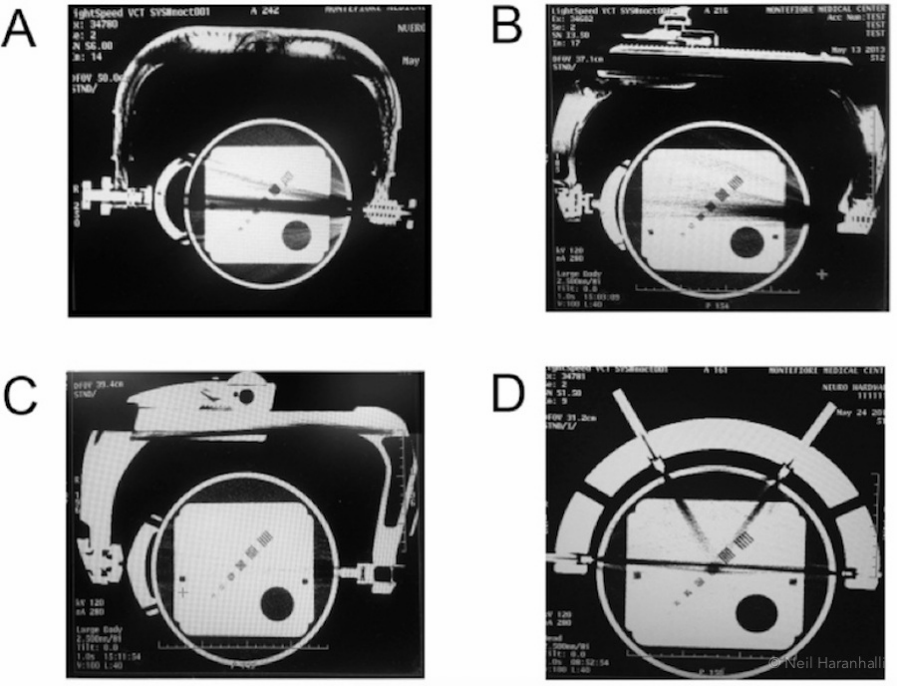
Four near-radiolucent frames in conventional CT Upper row (A and B), two Mayfield skull clamps (C). A radiolucent Doro skull clamp (D). A Sugita carbon fiber head frame. Note the dark streaks along the line of greatest attenuation originating from the metal objects adjacent to the pins
